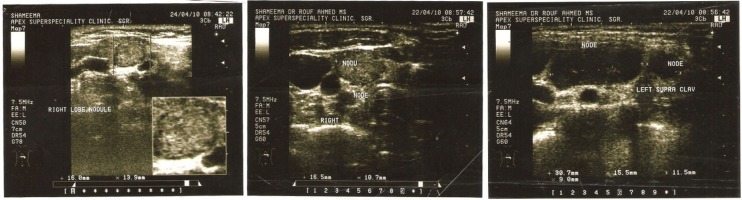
Ultrasonography of Neck Shows a Solid Thyroid Nodule. Ultrasound neck Showing a) Solid nodule in right lobe of thyroid b) Multiple enlarged right cervical lymph nodes c) Multiple enlarged lymph nodes in left supraclavicular region
